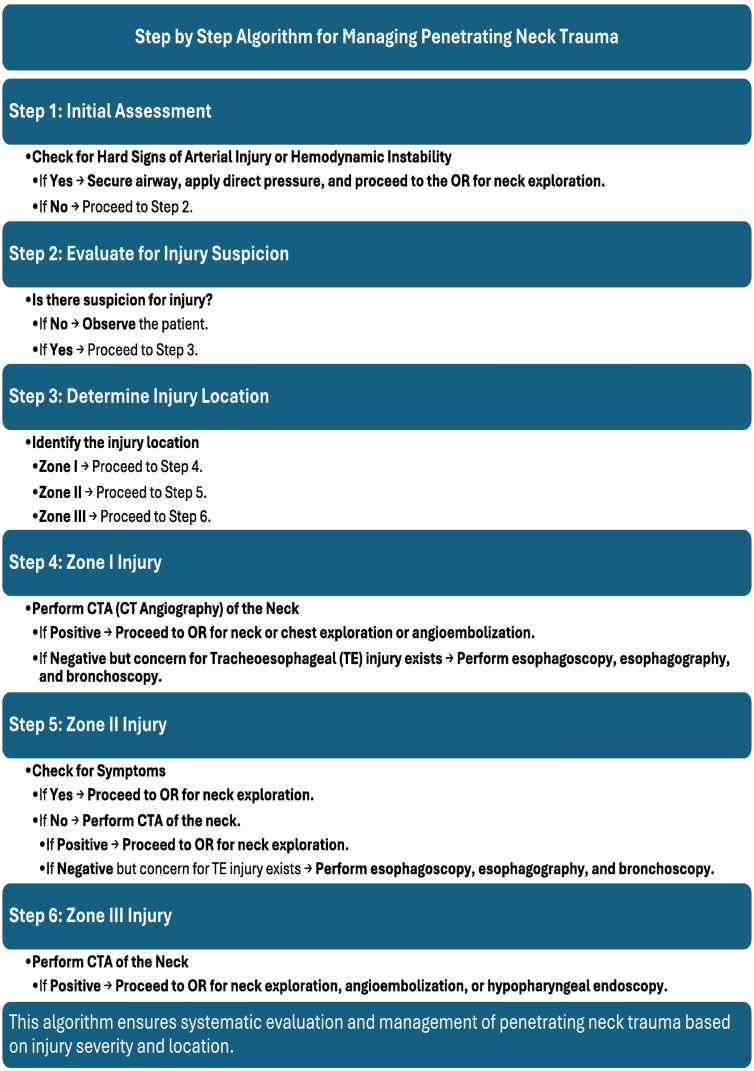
Algorithm for the management of penetrating neck injuries. Hard signs include active bleeding, expanding neck hematoma, air bubbling from the wound, neurologic deficit, and hematemesis. *CTA*, Computed tomography angiography; *OR*, operating room; *TE*, tracheoesophageal. (Modified from Sperry JL, Moore EE, Coimbra R, et al.: Western Trauma Association critical decisions in trauma: Penetrating neck trauma. *J Trauma Acute Care Surg* 75:936–940, 2013.) SOURCE: https://www.surgicalcore.org/popup/417026.
